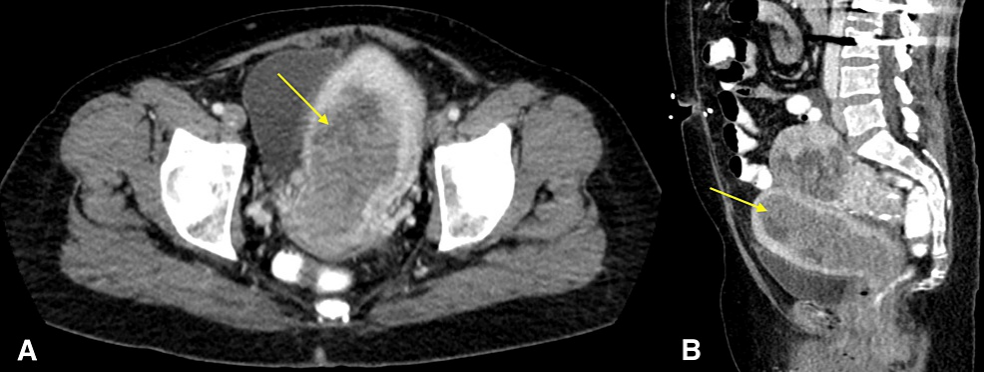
Computed tomography of the abdomen and pelvis with IV contrast in axial (A) and sagittal (B) views. There is marked lobular heterogeneous thickening of the central endometrium measuring up to 5.6 cm (yellow arrow). This mass extends from the uterine fundus to the endocervical canal and upper vagina.
